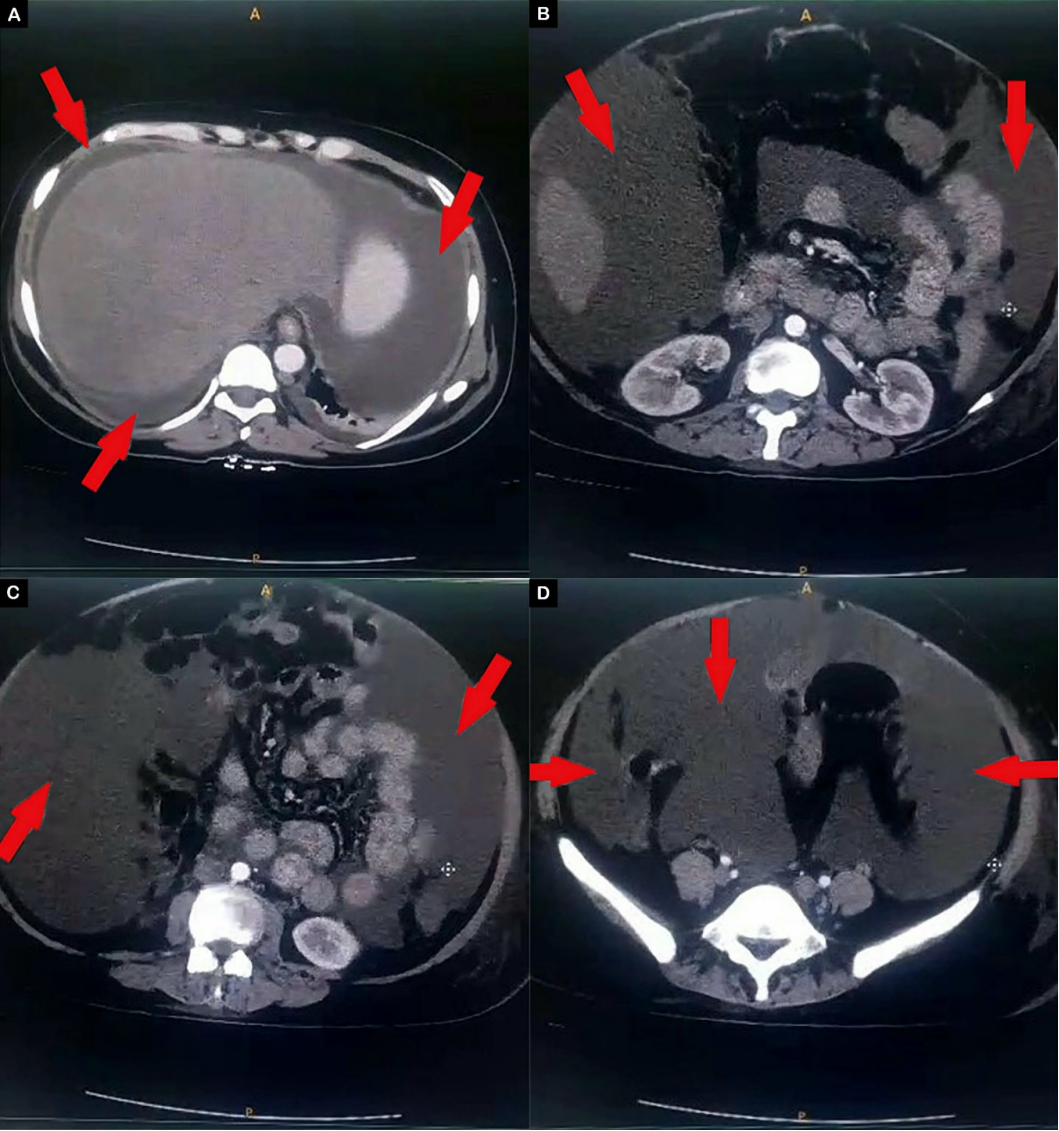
Axial computed tomography section of the abdomen showing massive ascites (red arrows). A At the T9–T10 level, at the superior aspect of the liver adjacent to the diaphragmatic, dome fluid can be observed, consistent with a subphrenic fluid collection. B At the T12–L1 level, free fluid is present between the bowel loops. C At the L1–L2 vertebral level. D At the L3–L4 vertebral level, at the region of the iliac wing
